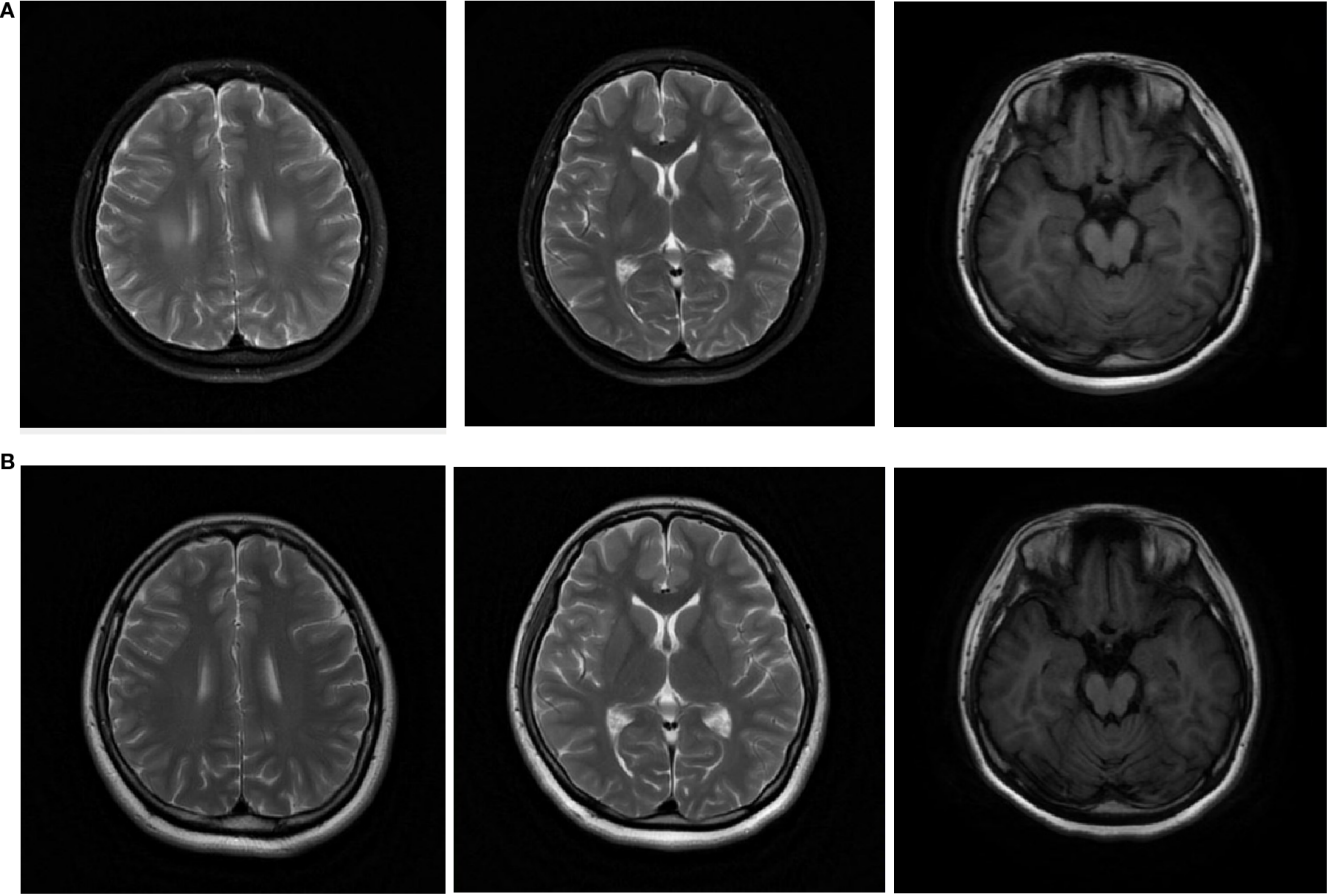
Brain MRI of a patient showed lesions in bilateral pyramidal tracts and semiovale centrum at disease onset (a). In the follow-up MRI conducted 17 months later, the lesions significantly improved while cerebellar atrophy were noticed (b). Left and middle: T2 sequence; right: T1 sequence.
